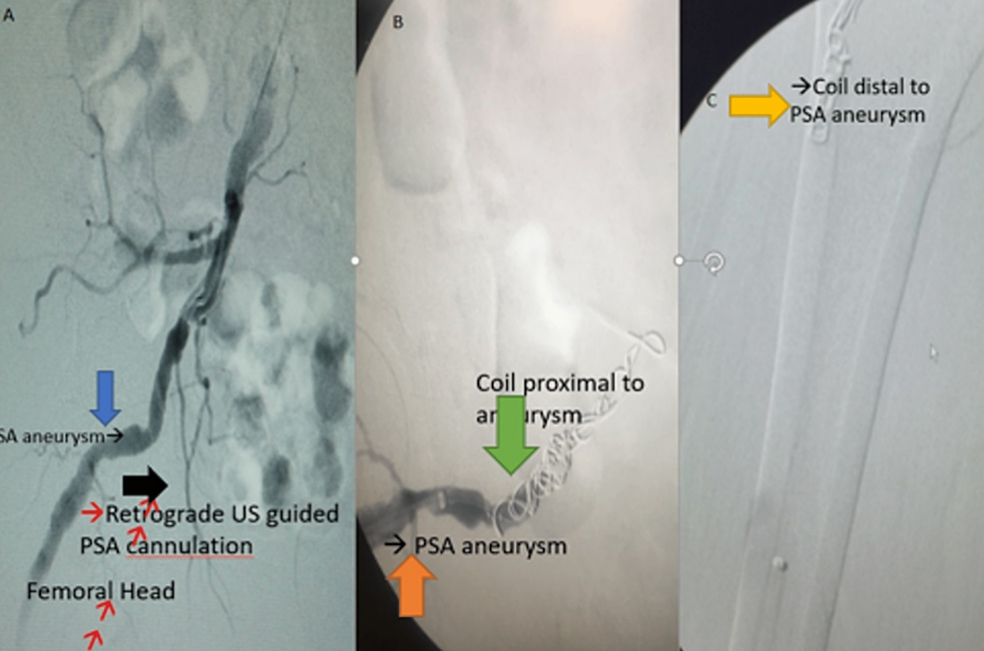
A: Persistent sciatic artery aneurysm (blue arrow), retrograde ultrasound-guided PSA cannulation (black arrow) B: PSA aneurysm (orange arrow); coil proximal to aneurysm (green arrow); C: Distal coil to PSA aneurysm (yellow arrow).
